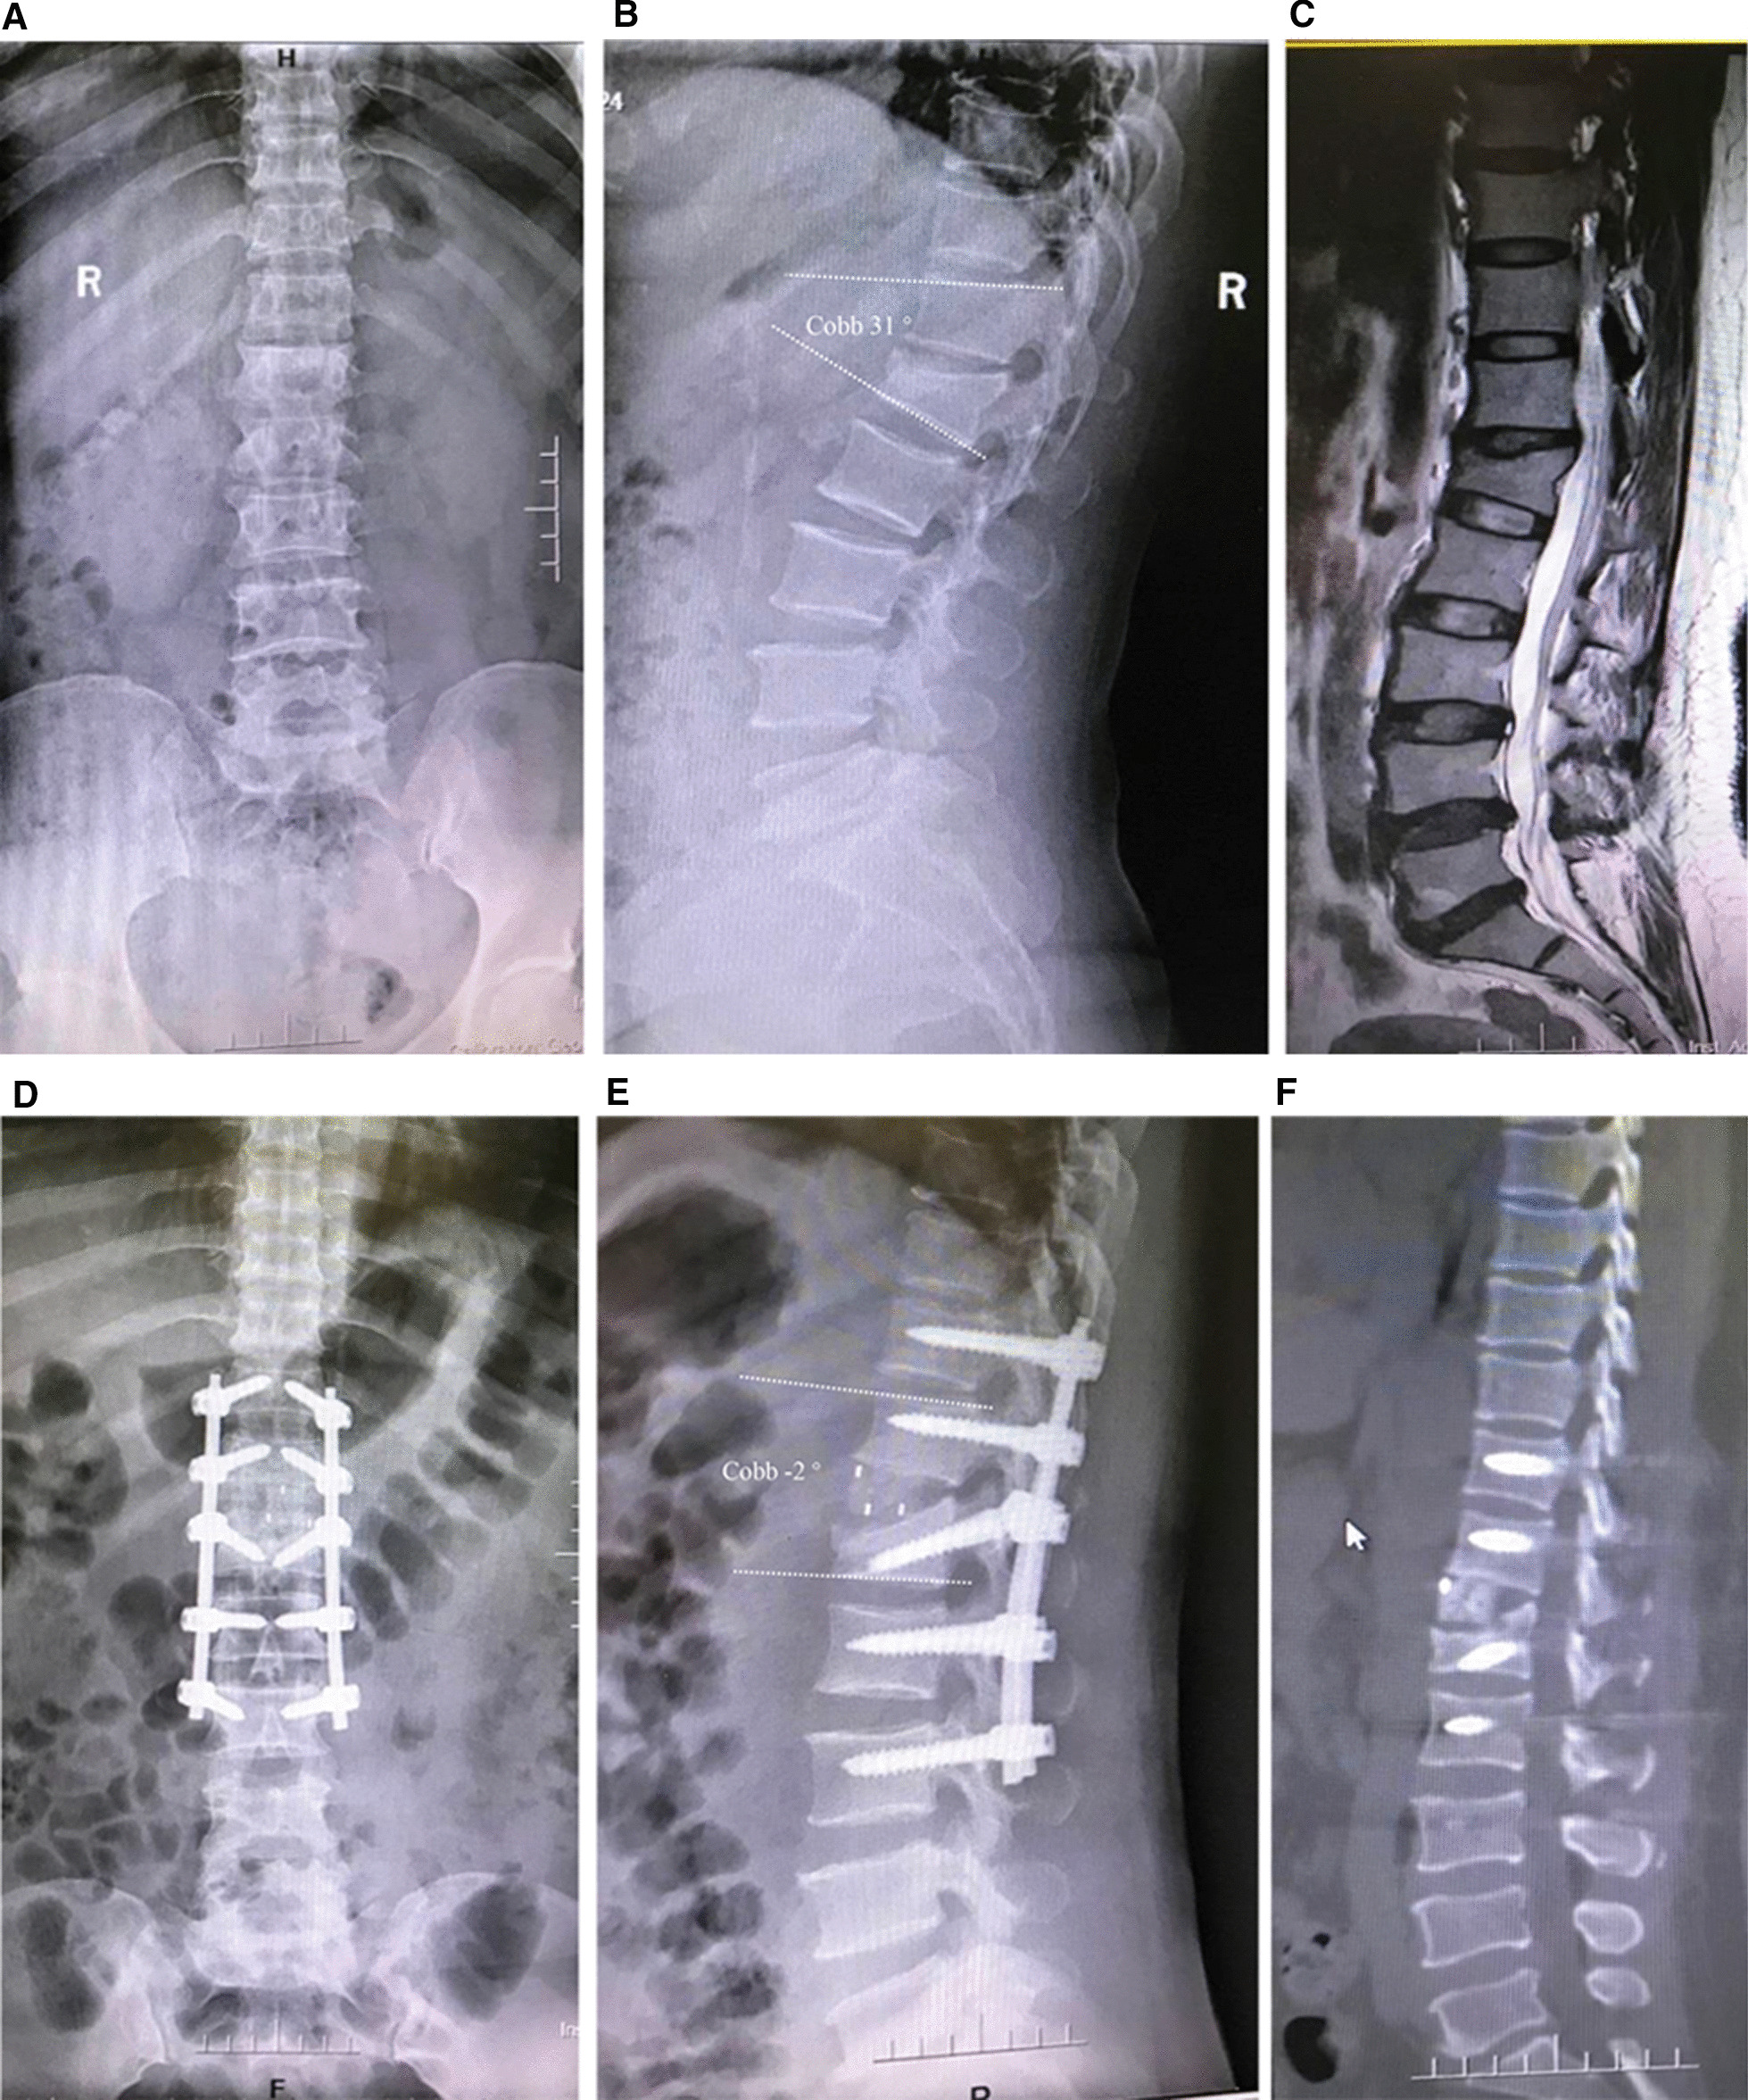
A 49 years-old female was diagnosed with posttraumatic thoracolumbar kyphosis. A, B The X-ray indicated that the L1 vertebra suffered from an old burst fracture with a local cobb angle of 22°kyphosis. C MRI showed compression of the dural sac and anterior displacement of the spinal conus. D, E X-ray showed that the opening of the anterior intervertebral space was good and the recovery of the spinal sequence was satisfactory two weeks after the operation. F CT showed that the spine sequence was good and the fixation was firm at the final follow-up
